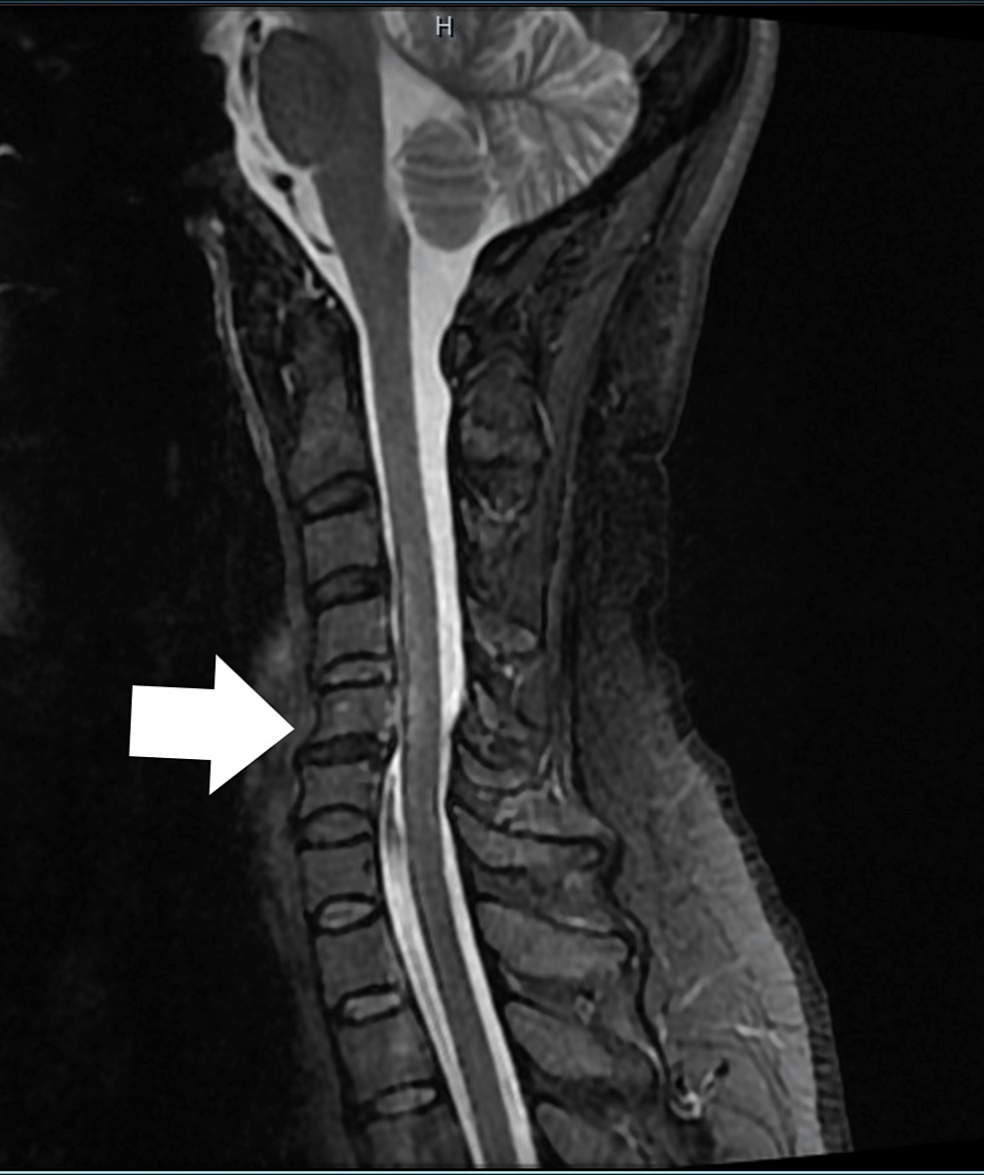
MRI of the cervical spine, sagittal T2 view showing degenerative disc changes in C5-C6 without cord compression
